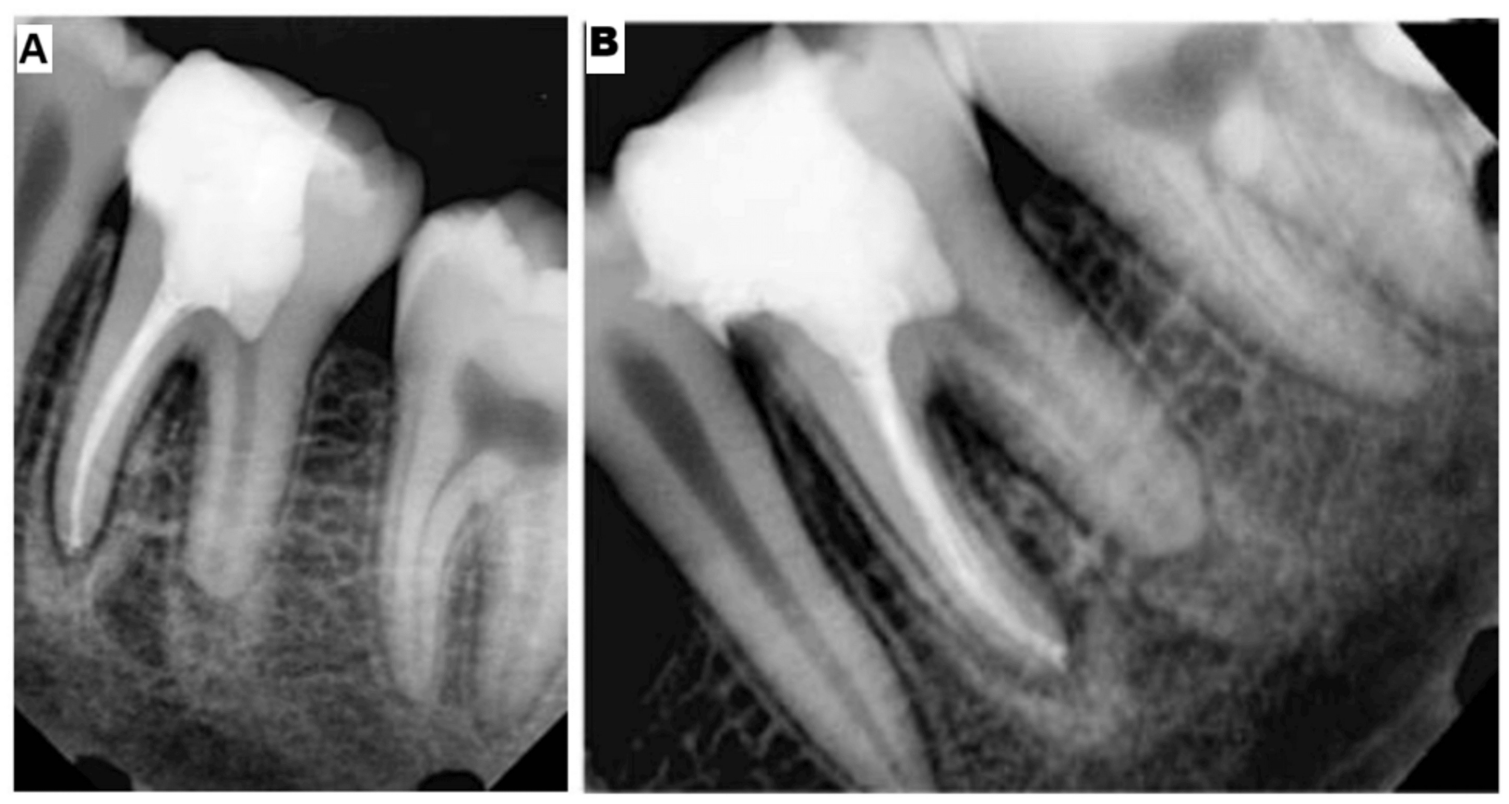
(A, B) Follow-up radiograph after one year
